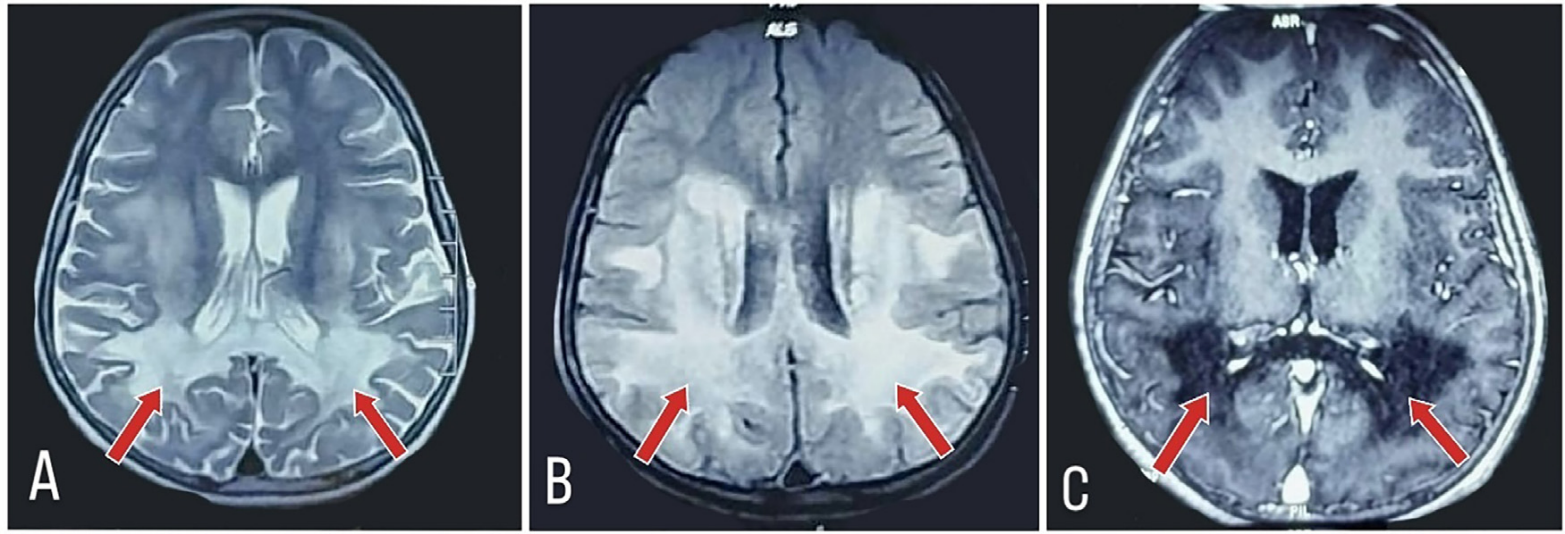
(A) MRI brain T2 weighted axial sections showing bilateral parieto-occipital white matter abnormal signals in periventricular and subcortical planes marked with red arrows. (B) MRI brain (FLAIR) axial sections showing hyperintensities in bilateral parieto-occipital regions with white matter abnormal signals in periventricular and subcortical planes marked with red arrows. (C) MRI brain showing no significant enhancement on post contrast scan marked with red arrows.
